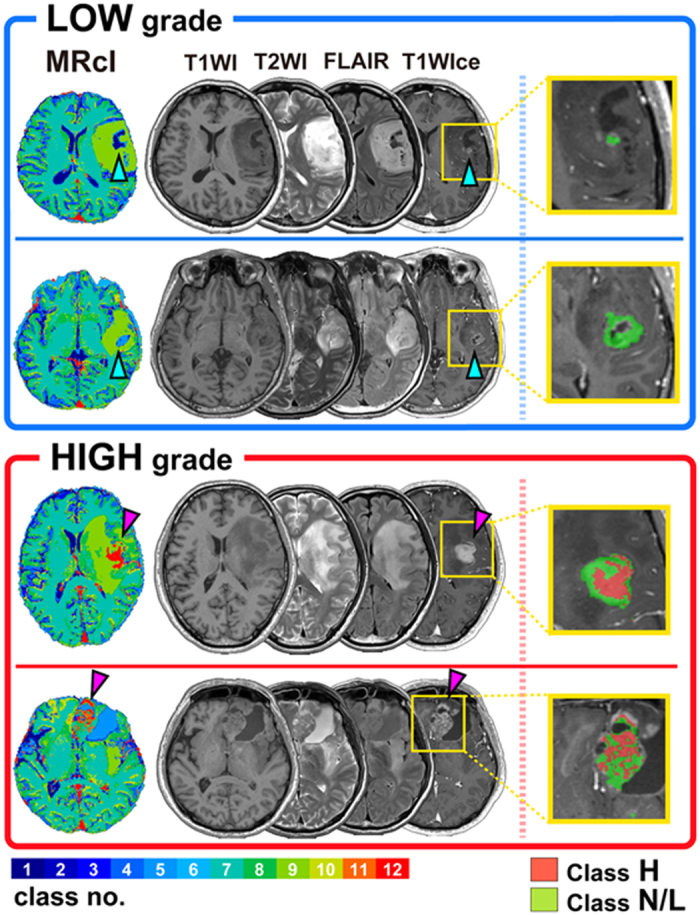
Representative cases of low- and high-grade gliomas, including the 12-class MRcIs, which showed the highest classification performance. The MRcIs, T1WI, T1WIce, T2WI, and FLAIR are shown for each patient. Each color on the MRcIs corresponds to each class in the 12-color bar (left hand corner). Inside the enhanced tumor regions, Classes H and L/N are shown in red and green, respectively, on the enlarged T1WIce (right).
